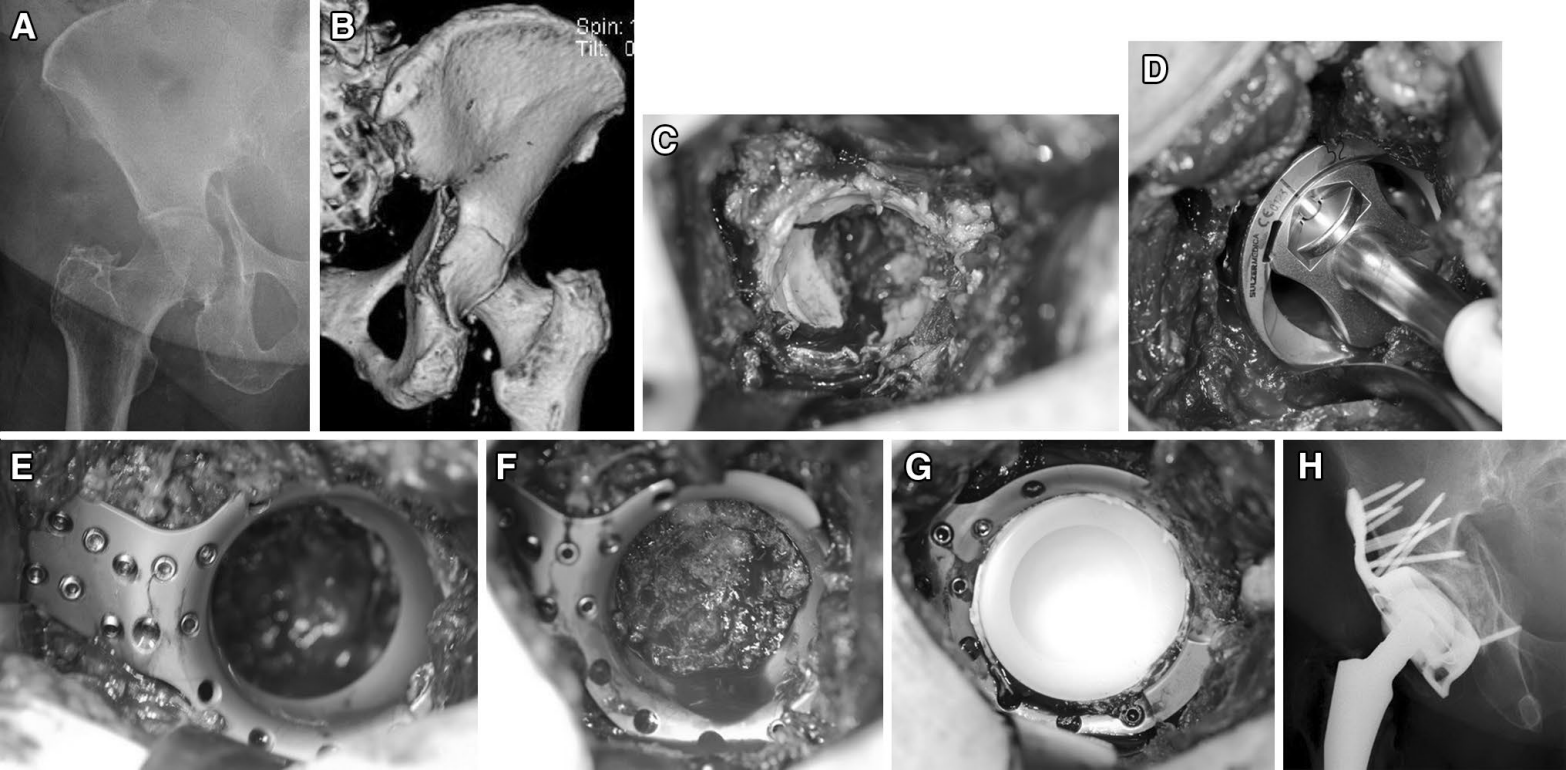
a Preoperative a.p. X-ray of a transverse acetabular fracture on the right side in a 79-year-old female. b Posterior view of a 3D-CT scan. c Intraoperative appearance of fracture. d Reaming of the fractured acetabulum up to 52 mm in diameter. e Custom-built Roof-Reinforcement Plate fixed with angle stable 3.5 mm screws. f Bone graft taken from the femoral head padding the cavity of the acetabulum. g Cemented 48 mm polyethylen inlay. h Postoperative a.p. X-ray
